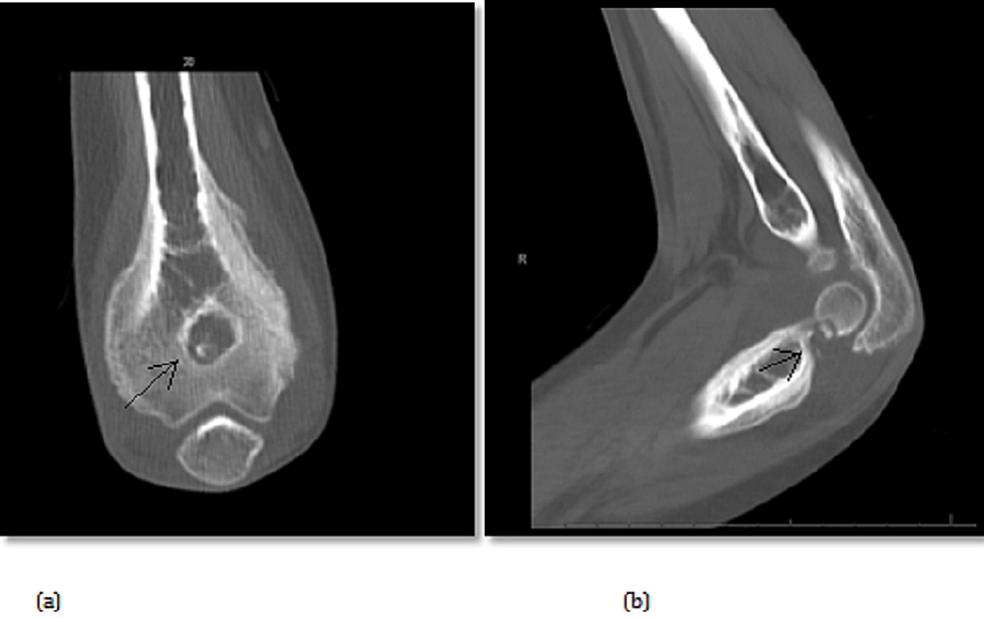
Preoperative computed tomography images [coronal (a) and sagittal (b), respectively] demonstrates a nidus comprising central sclerosis.
